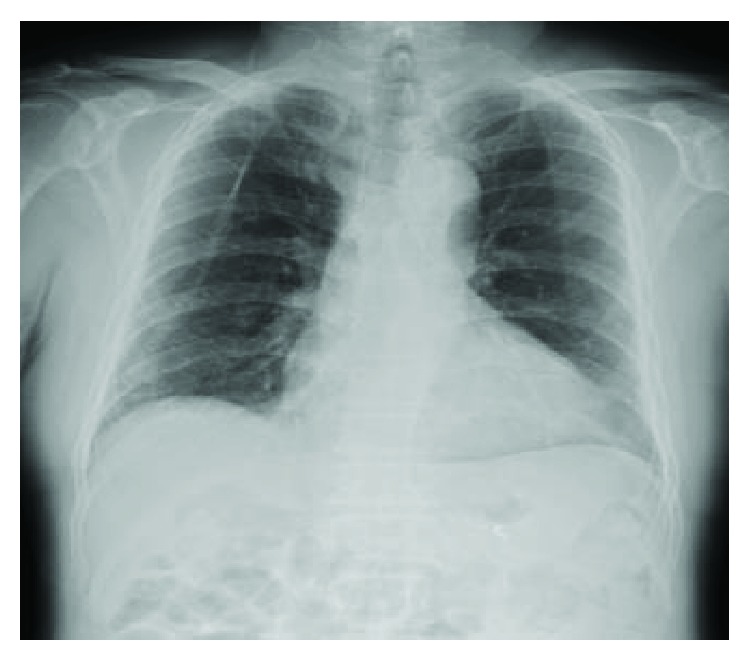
Chest X-ray examination in standing position does not reveal any free air.
